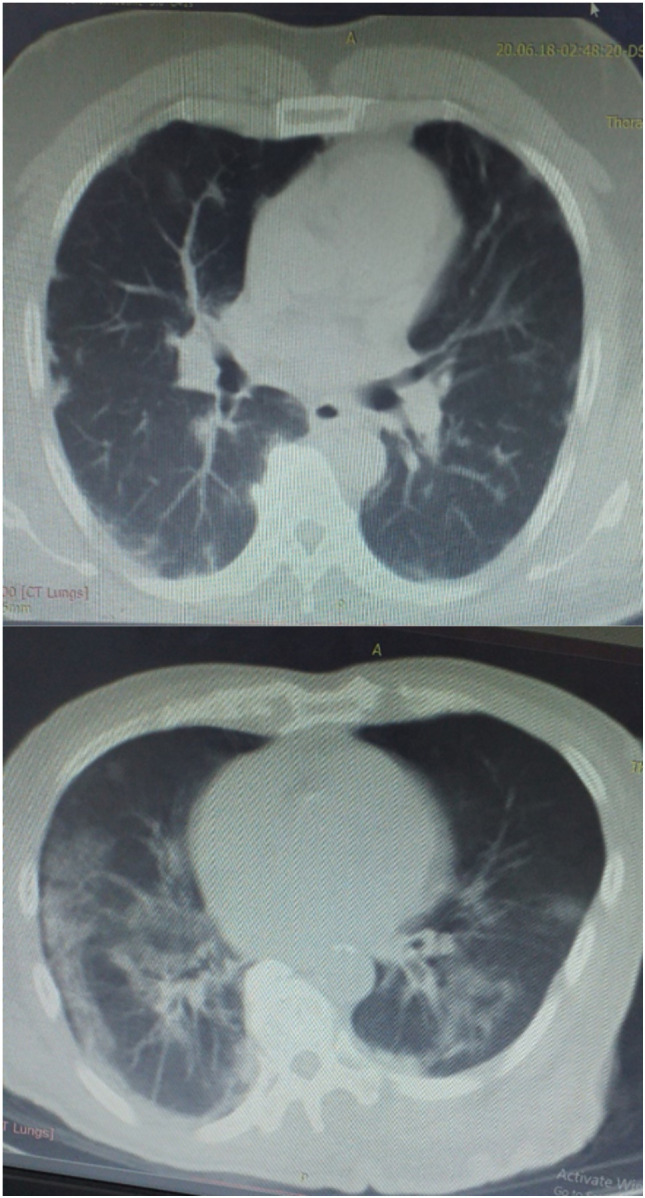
Unenhanced coronal CT images of confirmed COVID-19 cases showing the classical peripheral ground glass opacities in both lung fields
